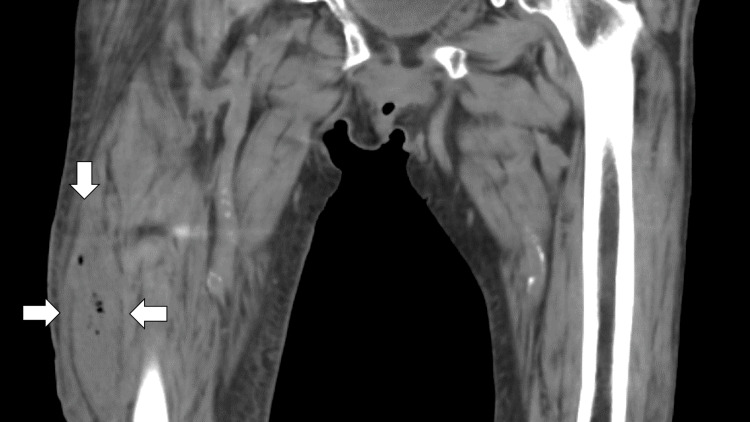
Femoral CT showing fluid retention and the included gas in the lateral part of the right thigh connecting with the previous surgical site of the femoral neck fracture (white arrows) CT: computed tomography
